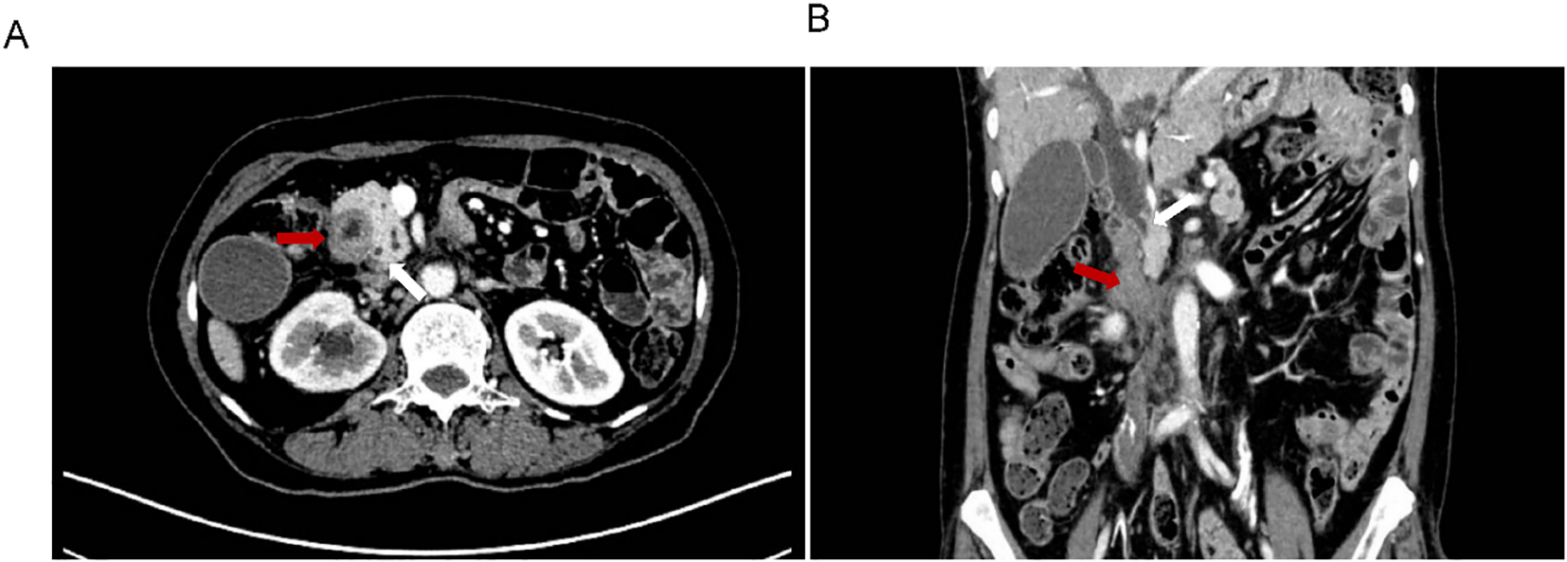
Preliminary PET-CT exam. The location of Duodenal lesions (red arrow) and bile duct obstruction (blue arrow) in coronal (A) and transverse (B) positions.
